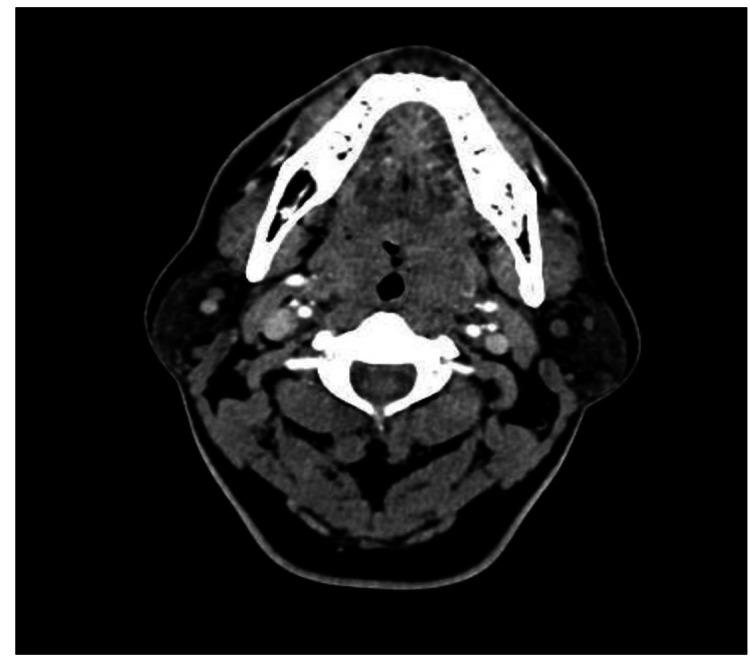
Contrast-enhanced neck CT of the patient Axial contrast-enhanced CT image shows an increase in the size of both tonsils, reducing the airways' light.
